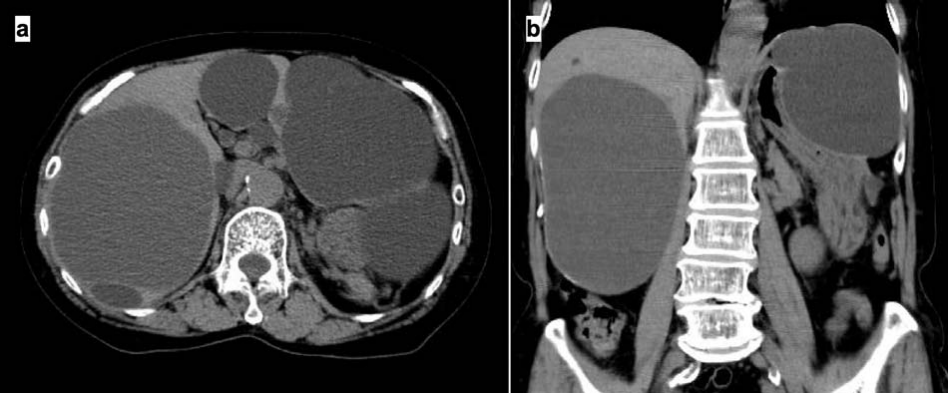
Preoperative CT imaging demonstrating multiple large liver cysts in the bilateral liver lobe and compression of the stomach due to a large cyst (a: axial section, b: coronal section).
